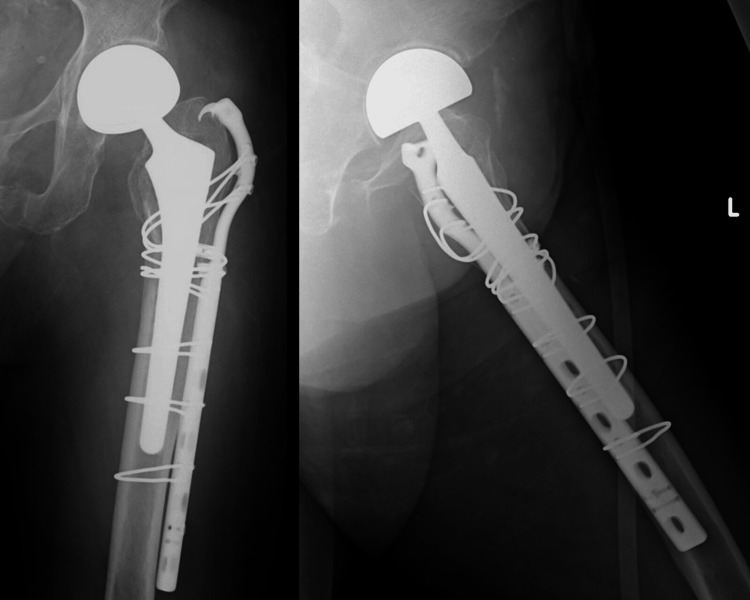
Postoperative radiographs of case 3 ORIF with plate fixation and cerclage wiring was performed. ORIF: open reduction and internal fixation.
